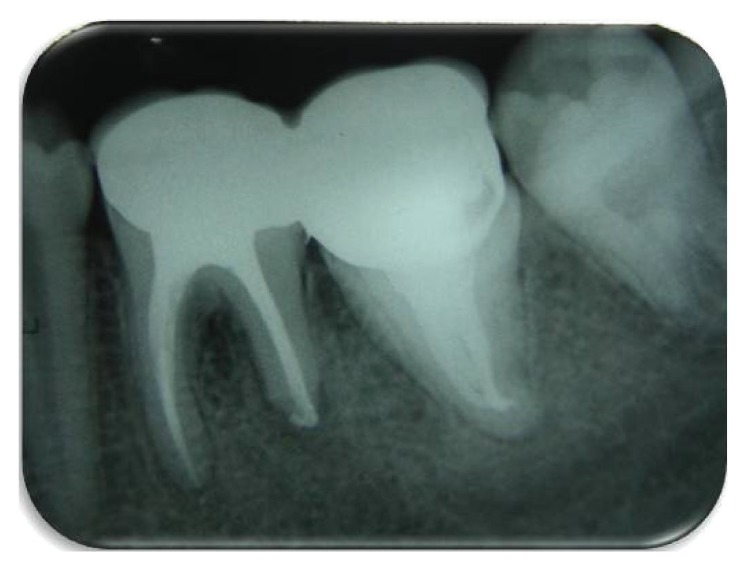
Recall radiograph 1-year postoperatively.
